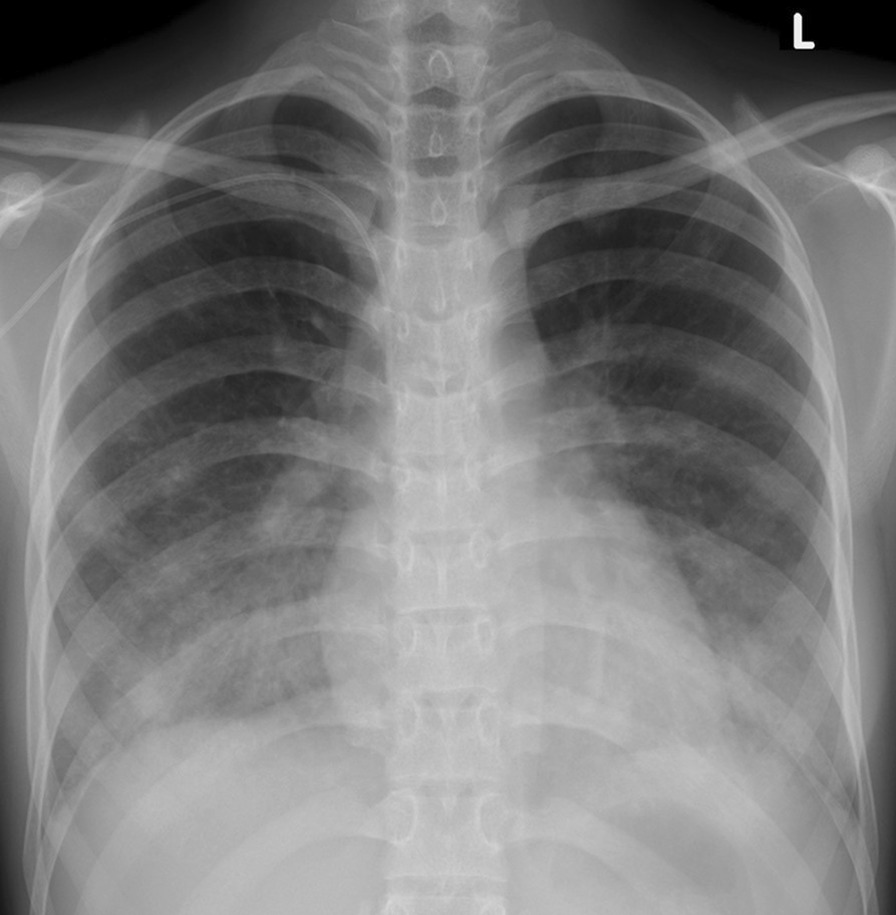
Follow-up chest radiograph showing a significant reduction of pulmonary alveolar opacities previously seen in bilateral middle and lower zones
